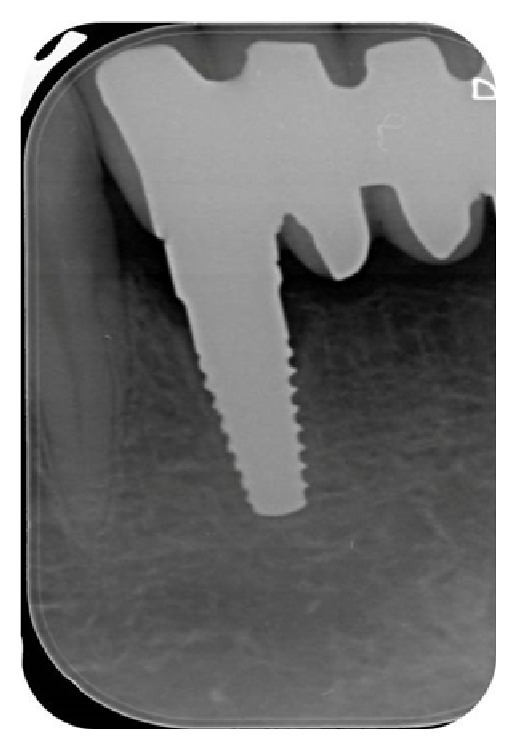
Radiographic control 4 years after implant insertions.
